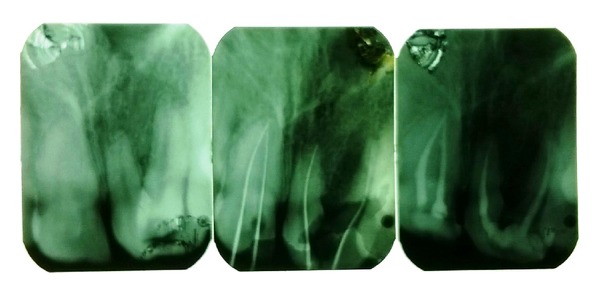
Intraoral periapical radiographs showing pre- and postendodontic treatment.
